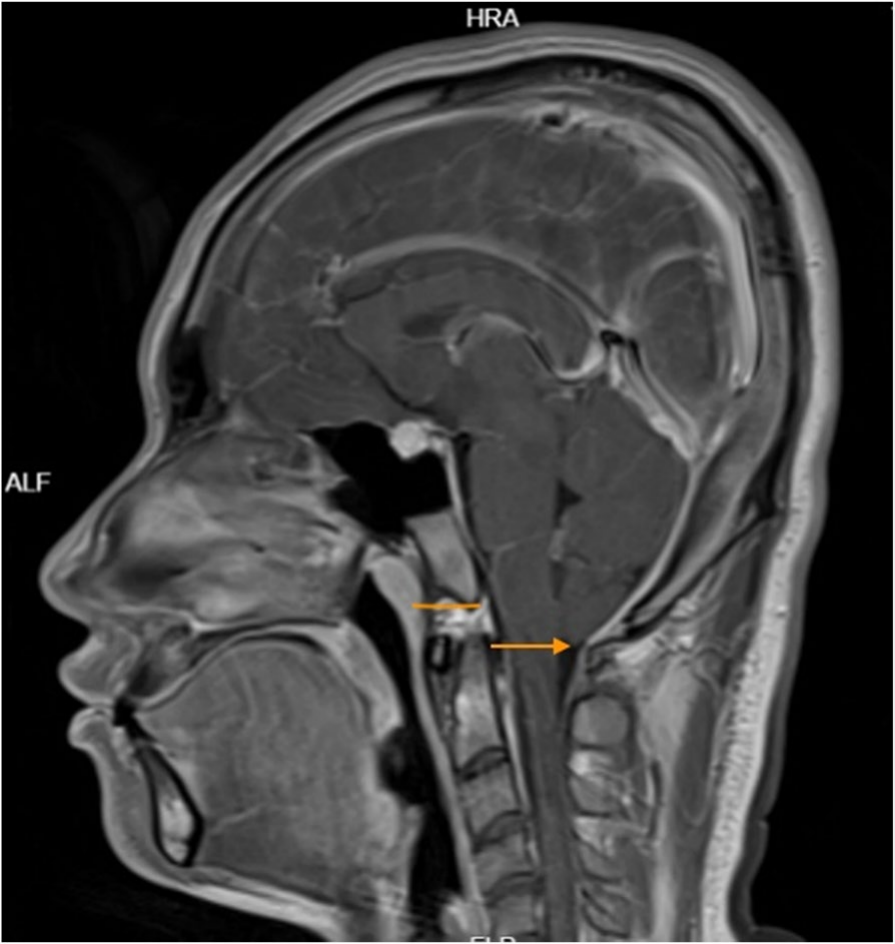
Contrast-enhanced brain MRI demonstrating cerebellar tonsillar herniation
